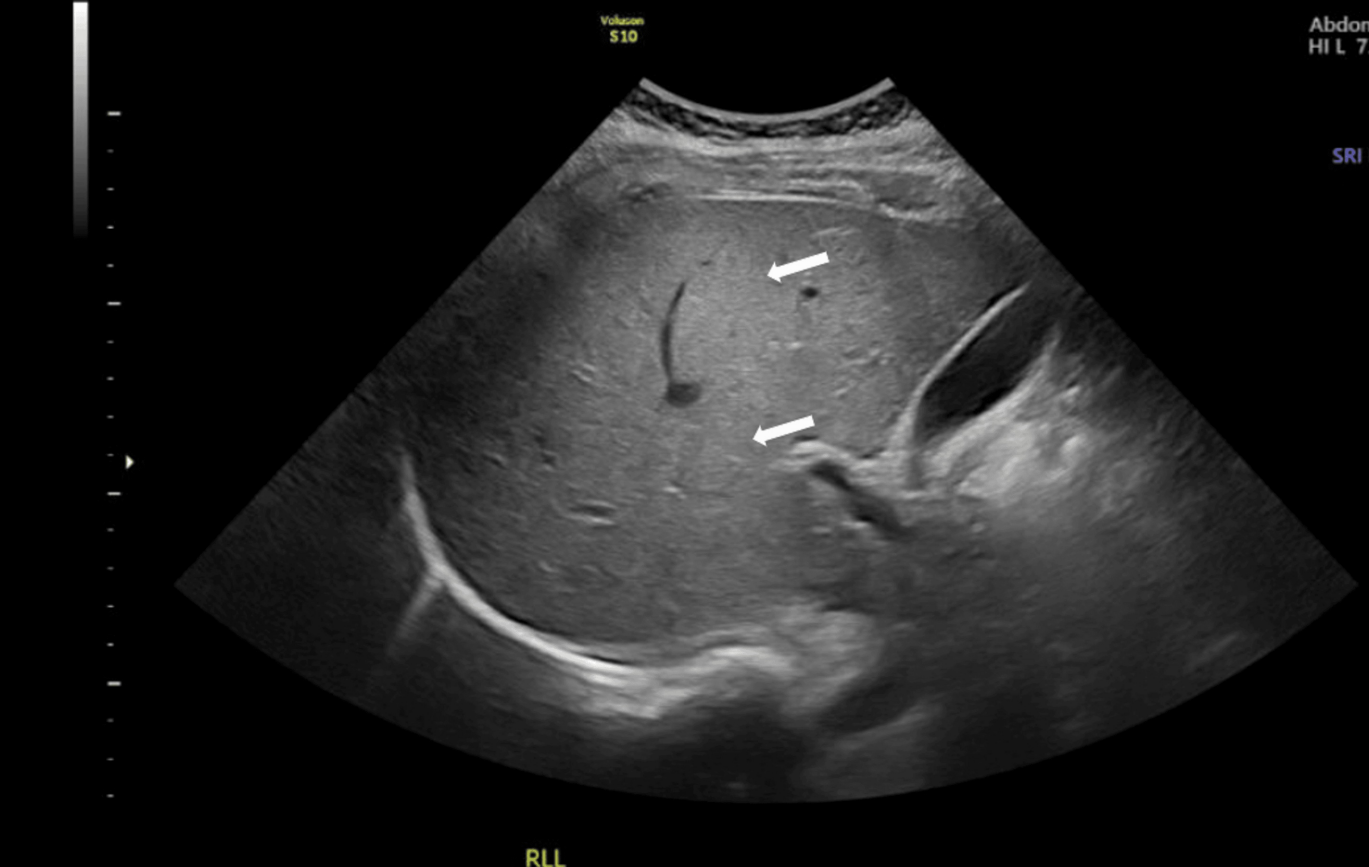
Ultrasound image of the patient’s liver. Ultrasound of the liver showing diffuse hyperechogenicity (white arrows) consistent with moderate hepatic steatosis.
